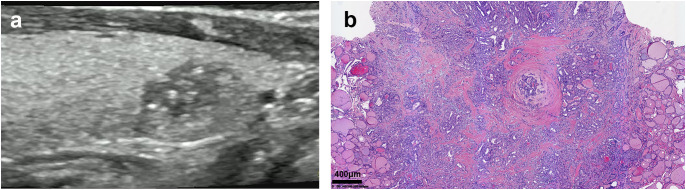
Ultrasound and pathological images of PTC without HT. (a) The parenchymal echo of the thyroid gland was homogeneous, and a hypoechoic nodule was non-homogeneous internal echo, and a dotted strong echo. (b) Pathology result: (HE, x40)Papillary thyroid carcinoma.
